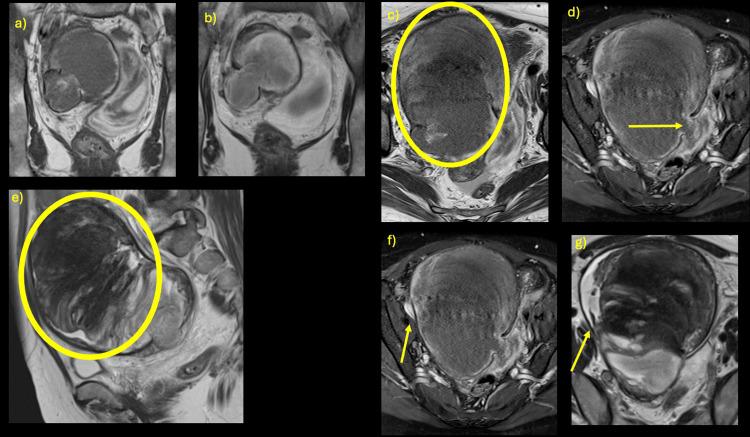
MRI two weeks after TAE (a, b) T1/T2-weighted images (axial view) showing a φ33 mm structure with no signal at the outer edge: first pseudoaneurysm (outer edge calcified). (c) T1-weighted image (axial view) showing a partially signal-free area that is torn off, and the structure has diffusion limitation equivalent to that within the mass from ventral to left (circle). (d) T1-weighted image with fat suppression (axial view) showing a high signal intensity outer edge: subacute hematoma (site of this rupture). (e) T2-weighted image (sagittal view) showing the ventral side of the aneurysm with a layered heterogeneous signal as if folded, with no enhancement, suggestive of an organized intra-aneurysmal thrombus (circle). (f, g) T1-weighted image with fat suppression and T2-weighted image (axial view) showing high signal intensity of the right margin of the mass, which suggests a residual, nonorganized area (arrow).
